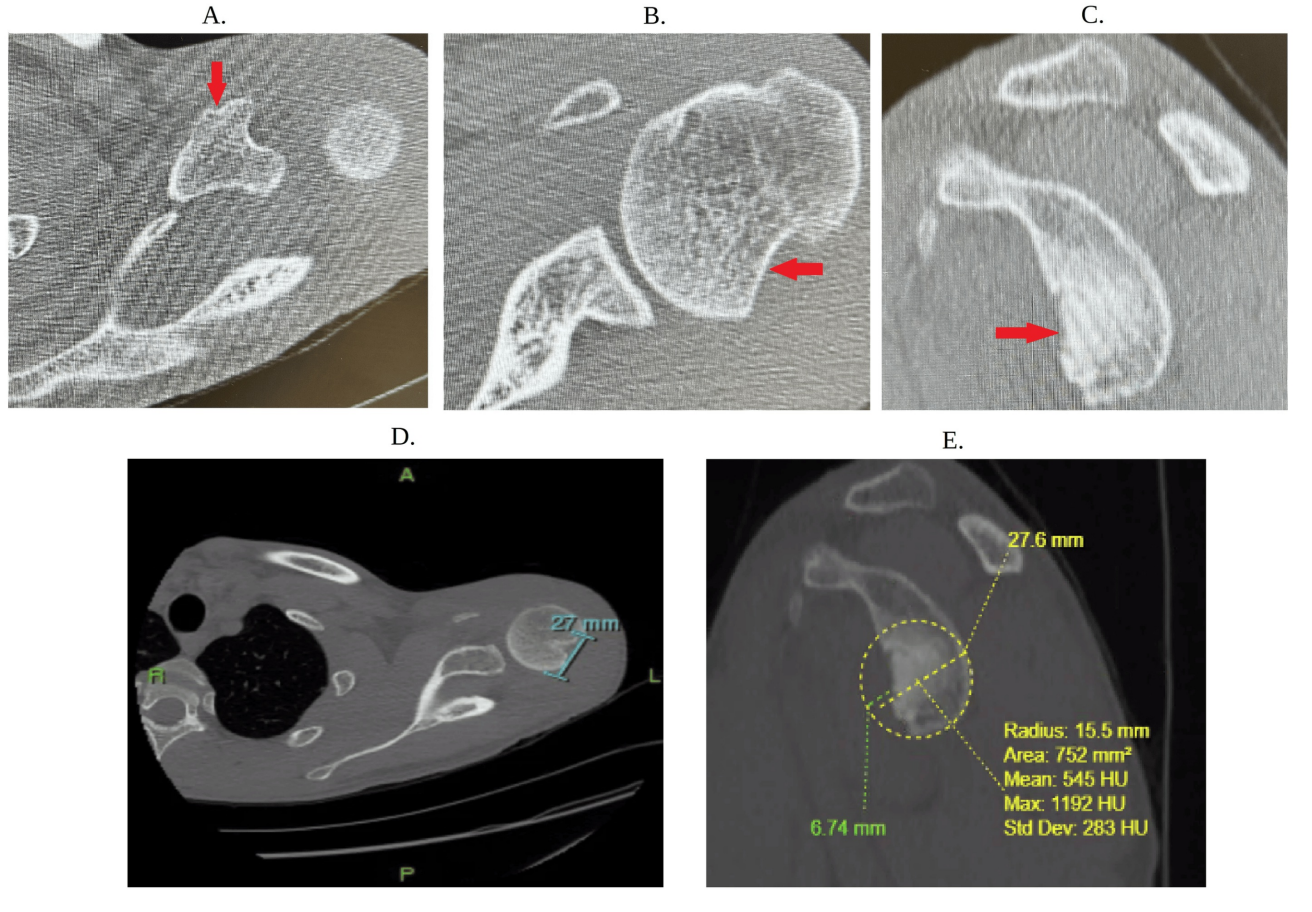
CT scan (a) The red arrow indicates the fractured coracoid. (b) The red arrow indicates the Hill-Sachs lesion. (c) This view is a sagittal CT view with the arrow showing bone loss. (d) Glenoid track measurement was based on the horizontal diameter of the glenoid, which is approximately 27 mm. The glenoid track calculation (0.83 × 27 mm)-6 mm shows a glenoid bone loss of approximately 16 mm. (e) Based on the measurement of the Hill-Sachs interval and glenoid track provided using the best-fit circle method in yellow, the Hill-Sachs interval is approximately 33 mm with magnetic resonance imaging without contrast in Figure 4 of approximately 24 mm, which is still greater than the glenoid track bone loss of approximately 16 mm compatible with an engaging off-track Hill-Sachs defect
